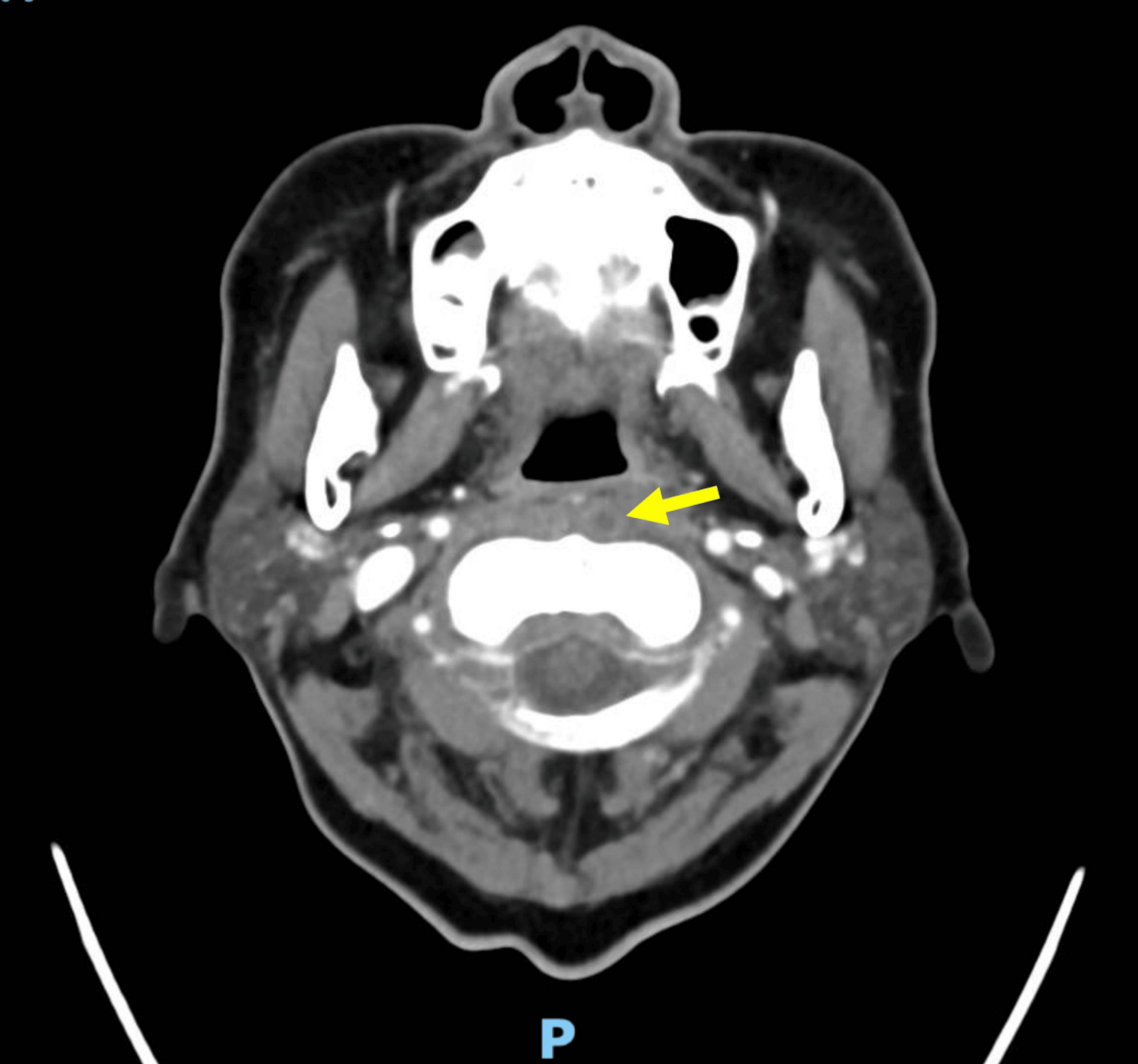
CT with intravenous contrast image of soft tissue of the neck (axial view) Rim-enhancing lesion measuring 8 mm in the left longus musculature at the C1-C2 level with associated retropharyngeal edema (yellow arrow). CT: computed tomography
